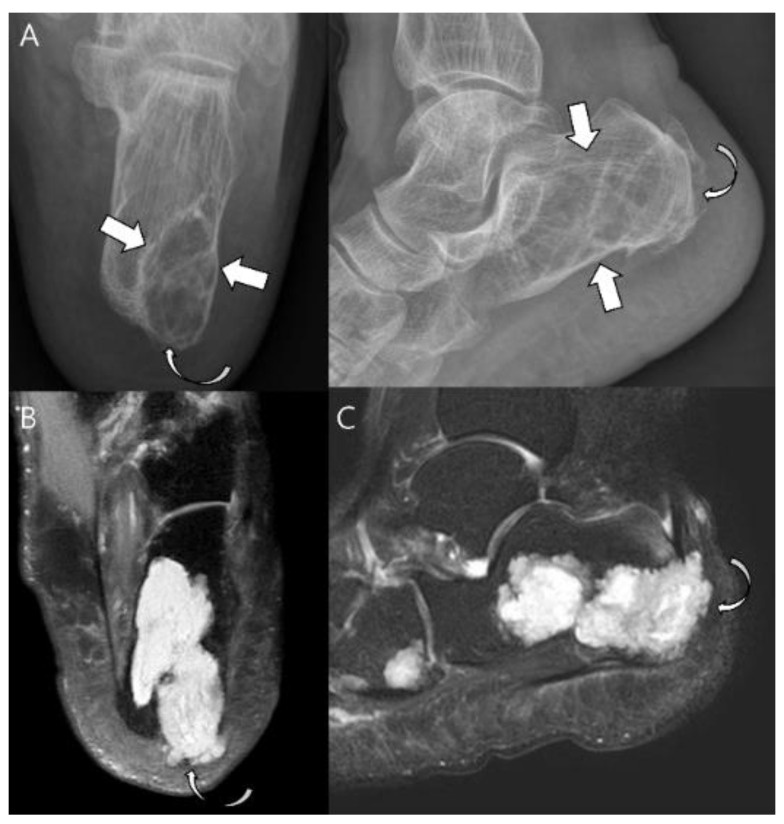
Atypical cartilaginous tumor of the calcaneus in a 74-year-old woman. (A) Plain radiographs reveal a lytic lesion in the calcaneus (arrows) with a partially destructed cortex (curved arrow). (B) Axial and (C) sagittal T2-weighted images with fat suppression show a lesion with marked high-signal intensity with focal extraosseous soft tissue extension (curved arrows).
